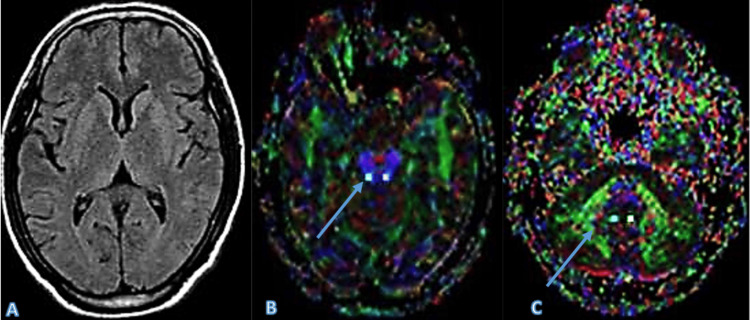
The T2-weighted image (A) appears normal with no obvious abnormalities. The DTI images (B, C), however, show a reduction in FA values in the superior cerebellar peduncle and dentate nucleus, which are regions implicated in essential tremor, providing critical diagnostic information that conventional MRI could not. Arrows in the DTI images indicate areas of FA reduction. DTI: Diffusion tensor imaging; FA: Fractional anisotropy
